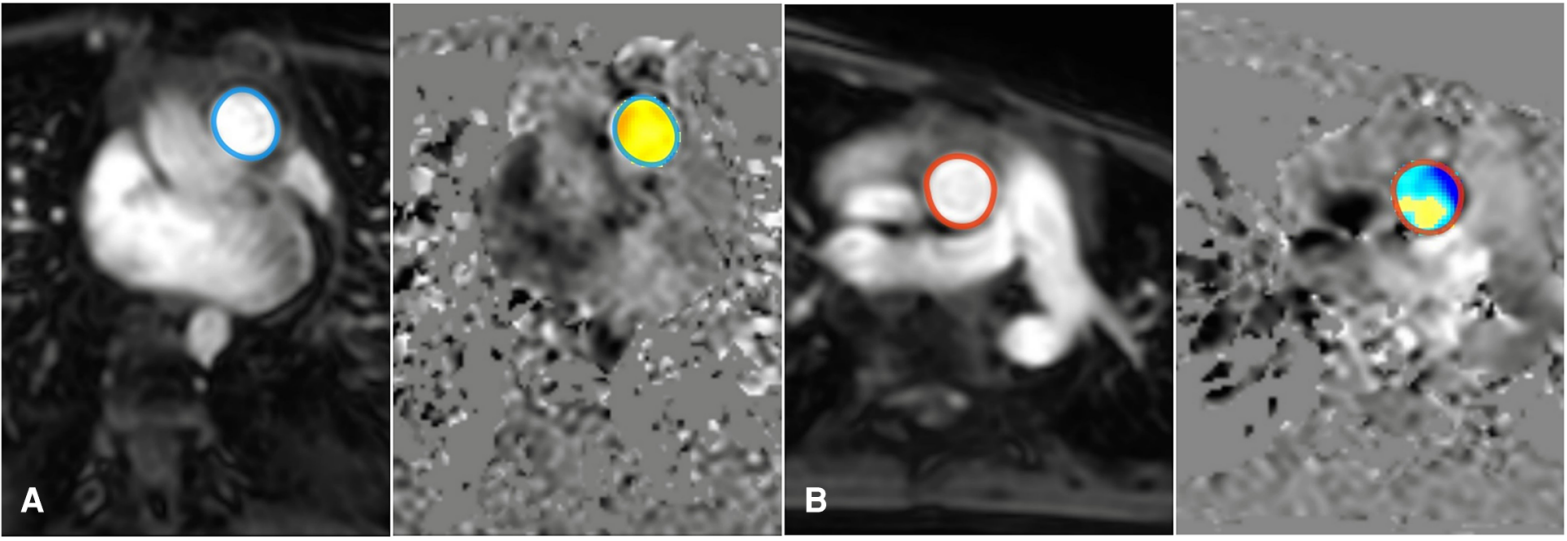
Measurement of the shunt fraction in 2D flow MRI: Add an ROI on the pulmonary artery (A), and ascending aorta artery (B).
